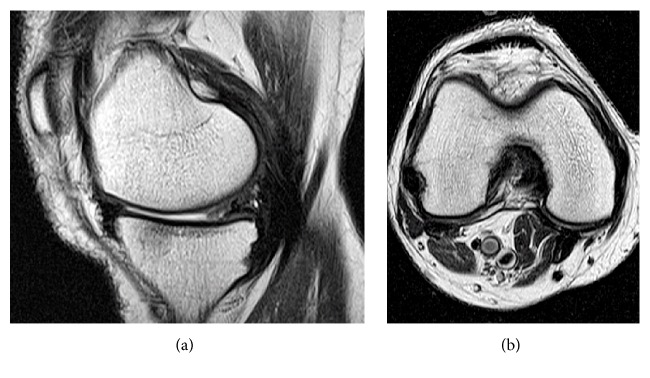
T2-weighted sagittal (a) and axial (b) magnetic resonance images taken 1 year postoperatively. No evidence of the popliteal cyst is observed.
